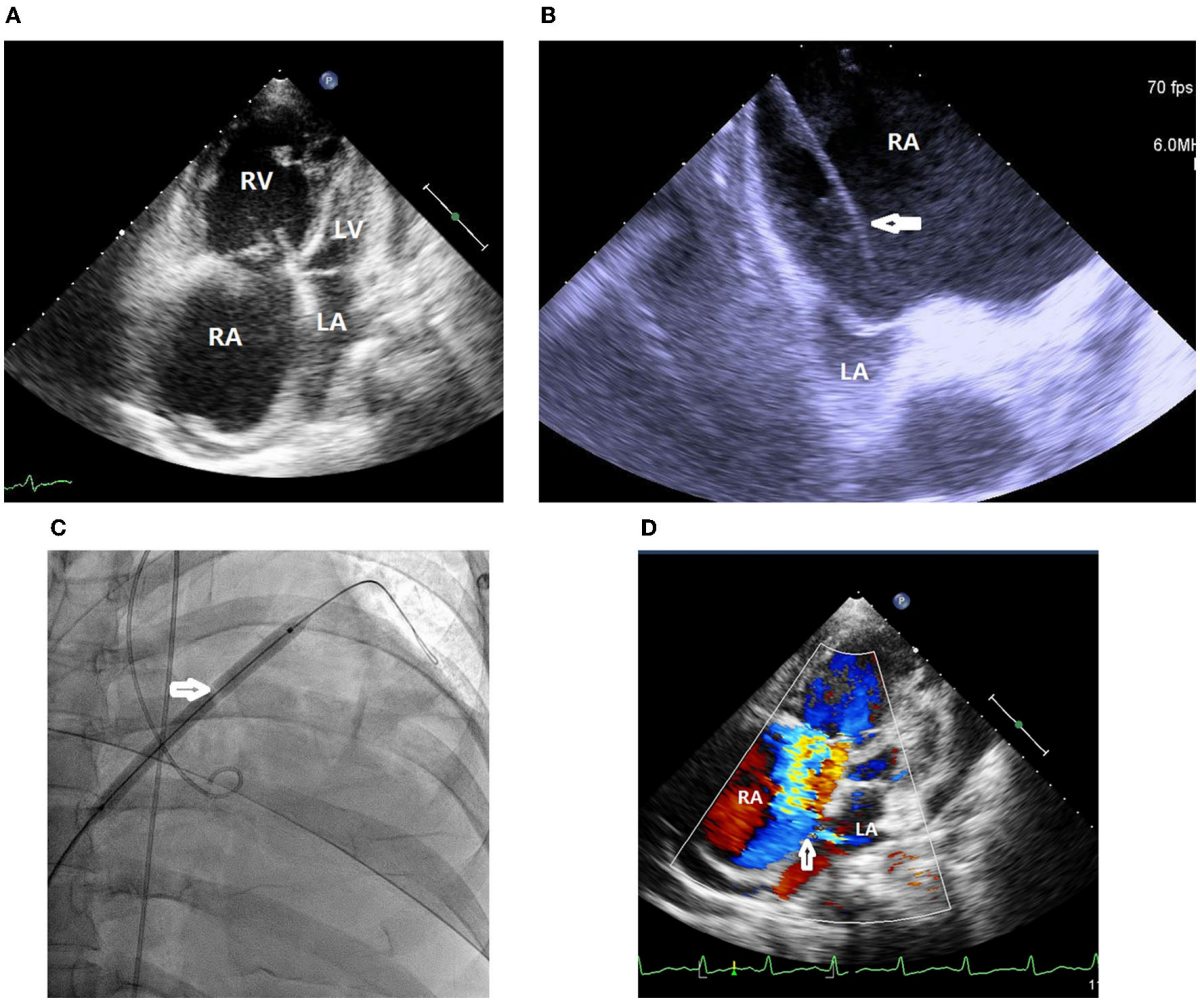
(A) Trans-thoracic echocardiogram revealed marked dilated right atrium and right ventricle in addition to small and compressed left atrium and left ventricle. RA, right atrium; RV, right ventricle; LA, left atrium; LV, left ventricle. (B) Intracardiac echocardiogram (ICE) showed marked dilated right atrium and small compressed left atrium. A transseptal Brockenbrough needle and a Mullins sheath (white arrow) was probing the inter-atrial septum with a tenting sign. RA, right atrium; LA, left atrium. (C) The atrial septum was dilated with a 6 mm × 8 cm balloon (white arrow). (D) Transthoracic echocardiogram confirmed the establishment of an inter-atrial shunt from right to left (white arrow). RA, right atrium; LA, left atrium.
